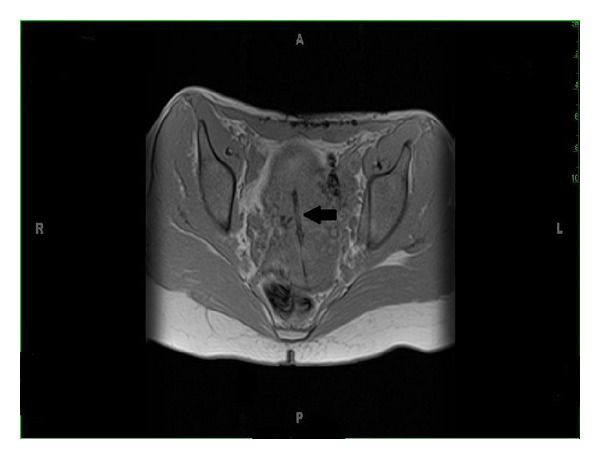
Arrow pointing to IUD within the uterus in a T1 weighted MRI, axial view.
